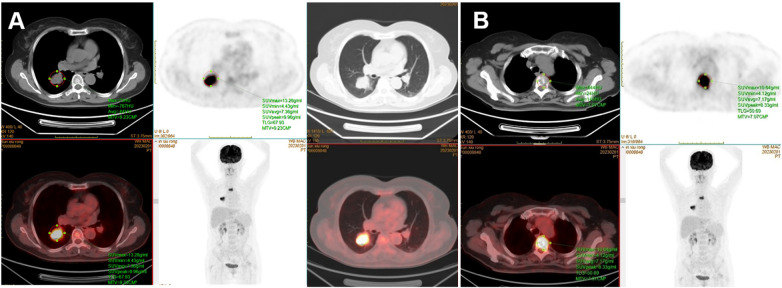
Representative ^18^F-FDG PET/CT images of a 66-year-old woman with EGFR 19Del-positive lung adenocarcinoma (cT4N0M1c, stage ⅣB). (A) Primary tumor in the right lower lobe: SUV_max_=13.28, SUV_min_=4.43, SUV_avg_=7.36, SUV_peak_=9.96, TLG=67.93, MTV=9.23 cm^3^. (B) Sternal metastasis: SUV_max_=10.64, SUV_min_=4.12, SUV_avg_=7.17, SUV_peak_=8.33, TLG=50.69, MTV=7.07 cm^3^.
